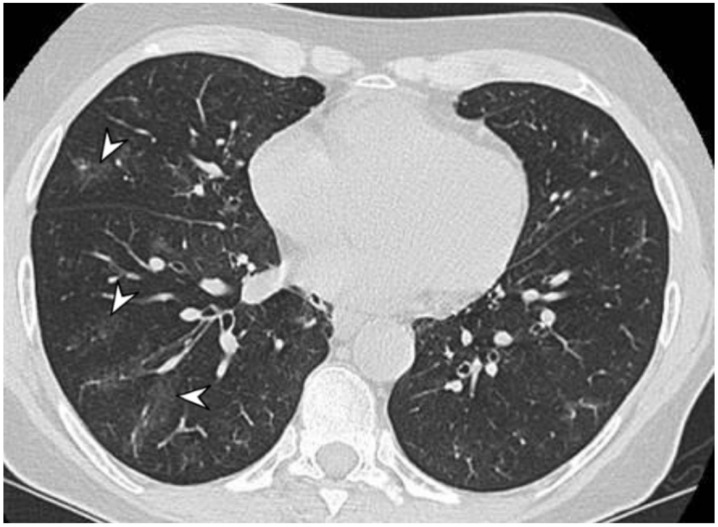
A 57-year-old female with p-ANCA associated vasculitidis. Axial high-resolution CT shows patchy ground-glass opacities (arrowheads), predominantly located in the right lower lobe, sparing subpleural regions.
